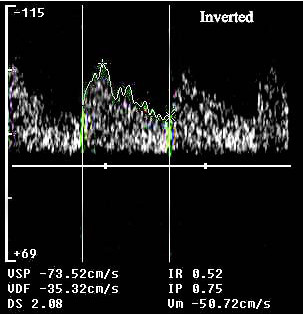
On the following day, the transcranial Doppler showed a normal flow at the level of the basilar artery: a low-resistance antegrade flow with mean flow velocity <60 cm/sec.
